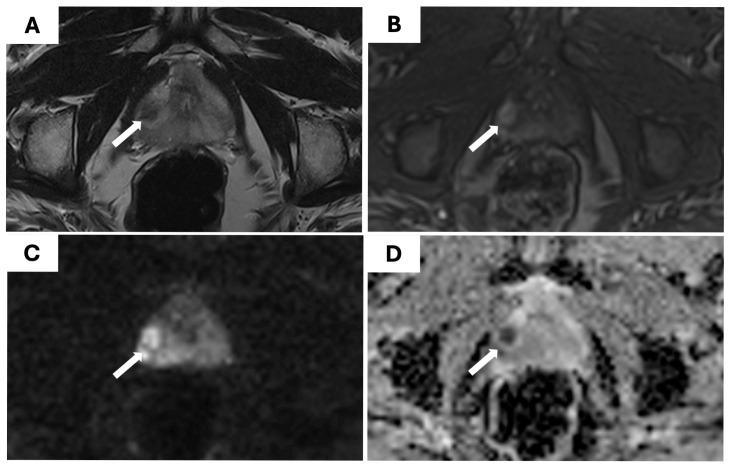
Representative case of a PI-RADS 4 lesion (white arrows): (A) axial T2-weighted MRI showing a hypointense lesion in the right peripheral zone; (B) dynamic contrast-enhanced image showing early enhancement; (C) diffusion-weighted imaging (DWI) b1500 revealing high signal intensity in the same region; (D) apparent diffusion coefficient (ADC) map showing marked hypointensity, consistent with restricted diffusion. The lesion was classified as PI-RADS 4 and confirmed as ISUP grade 2 adenocarcinoma on targeted and systematic biopsies.
